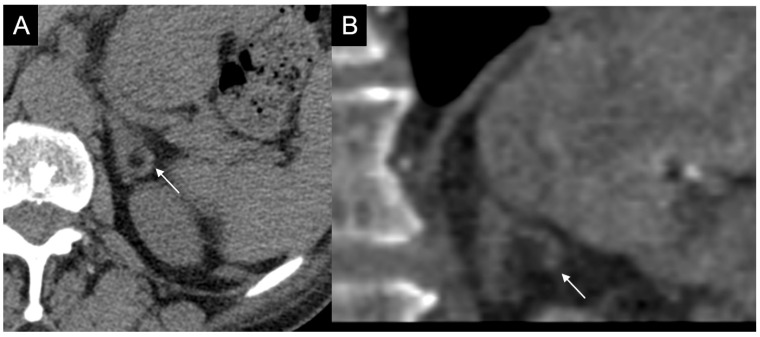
Doubtful hypodense nodule (arrow) inside the left adrenal gland on basal CT (A); in coronal reconstructions the adrenal gland has normal morphology (B).
